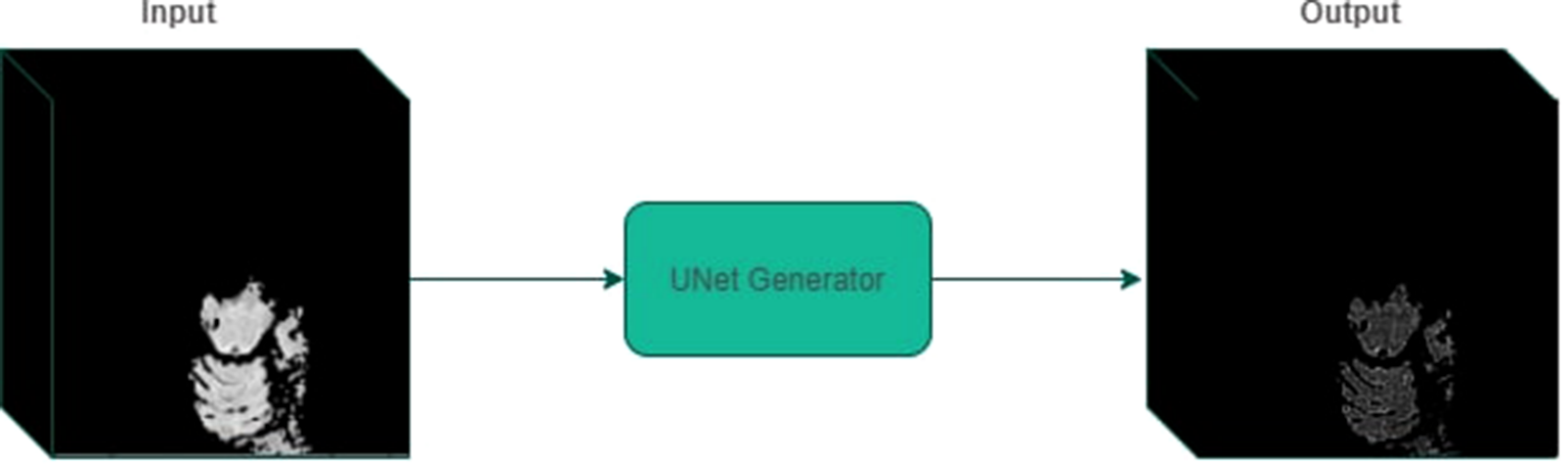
An example of perturbed mask generation using UNet generator. Given a 3D volume containing a segmented superpixel, the generator model outputs a mask that can be applied to the original MRI volume.
